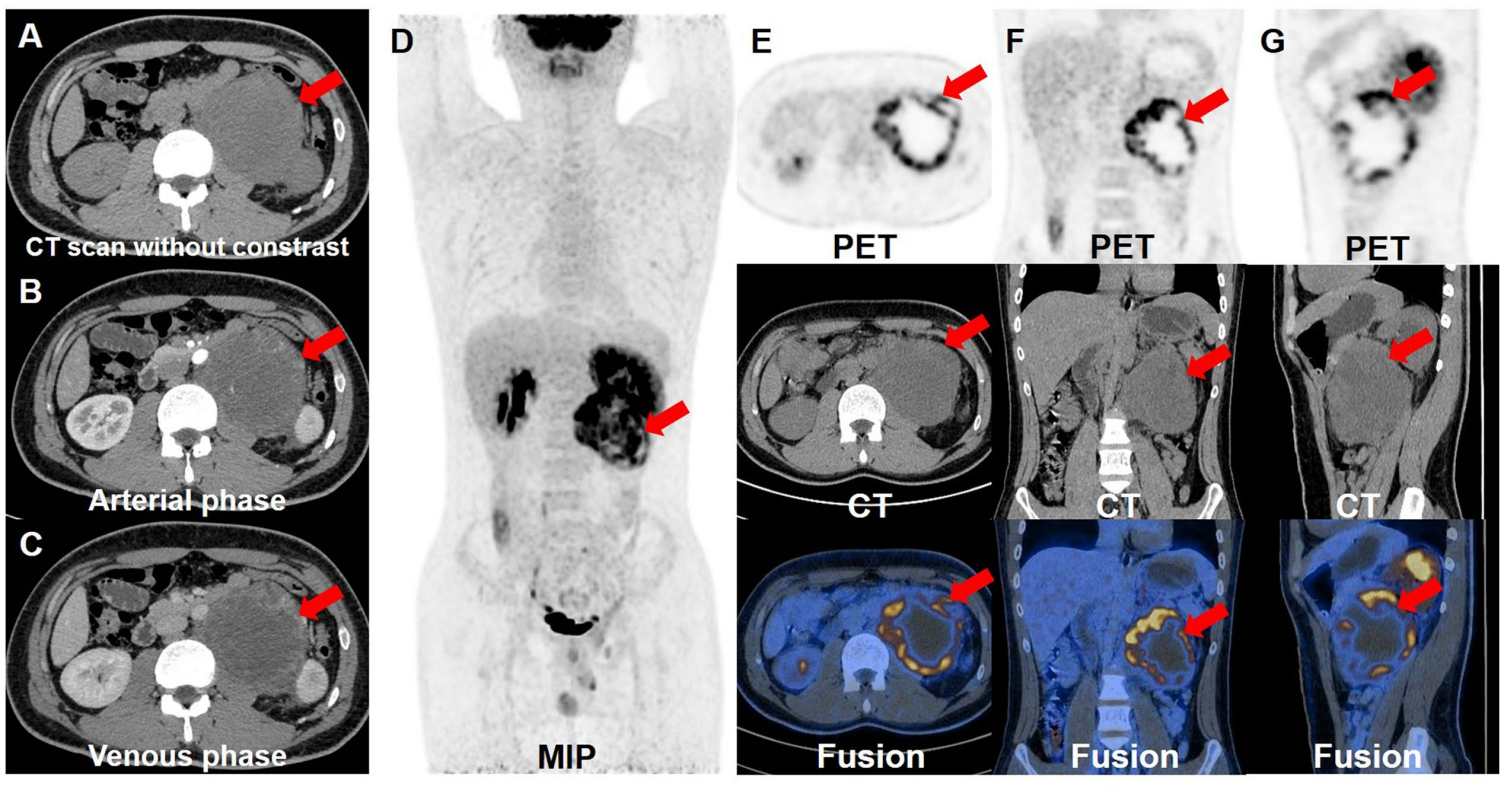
Contrast-enhanced CT and ^18^F-FDG PET/CT images. The retroperitoneal teratoma exhibited an incomplete capsule and unclear local boundaries on the enhanced CT plain scan (A), with uneven internal density. And it demonstrated uneven enhancement during the arterial (B) and venous (C) phases of the contrast-enhanced CT. (D) The whole-body maximum intensity projection showed the retroperitoneal lesion with hypermetabolism. The transverse (E), coronal (F) and sagittal (G) images of retroperitoneal lesions showed that the tumor had high FDG uptake, with a SUVmax of 15.9.
